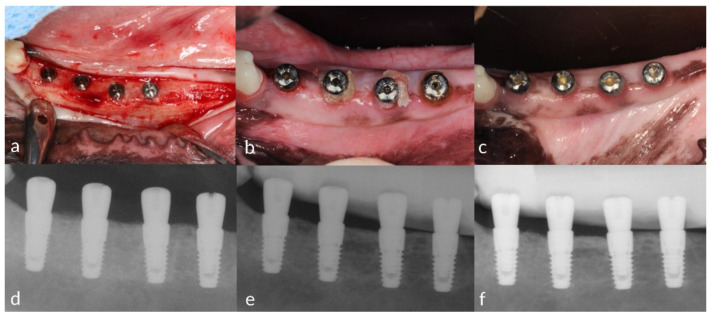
Clinical photographs and radiographs—Clinical photographs taken at (a) the surgery (S0), (b) the first drug administration (baseline, T1) and (c) 8 weeks after the baseline (T3). Peri-apical radiographs taken at (d) the surgery, (e) the baseline and (f) 8 weeks after the baseline.
